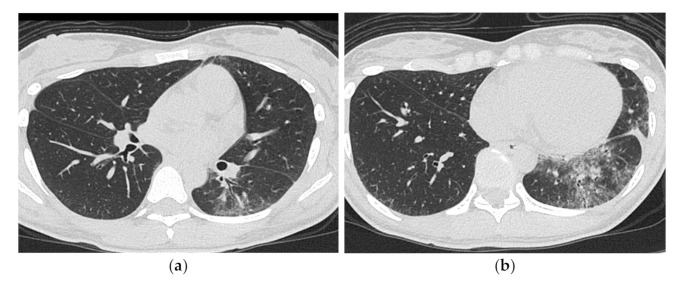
A 30-year-old man with nonspecific interstitial pneumonia pattern associated with dermatomyositis (anti-ARS Ab positive). HRCT images (a,b) shows bilateral ground-glass opacification and reticulation with peribronchovascular predominance. Mild traction bronchiectasis and decreased volume in the left lower lobe was also seen.
